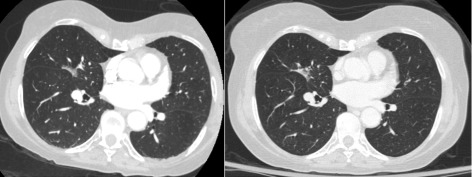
Slices from chest CT scans acquired four years prior to (left) and at the time of presentation (right), demonstrating an indolent, subsolid lesion in the right middle lobe, the solid fraction of which evolved from 15% (left) to 90% (right)
